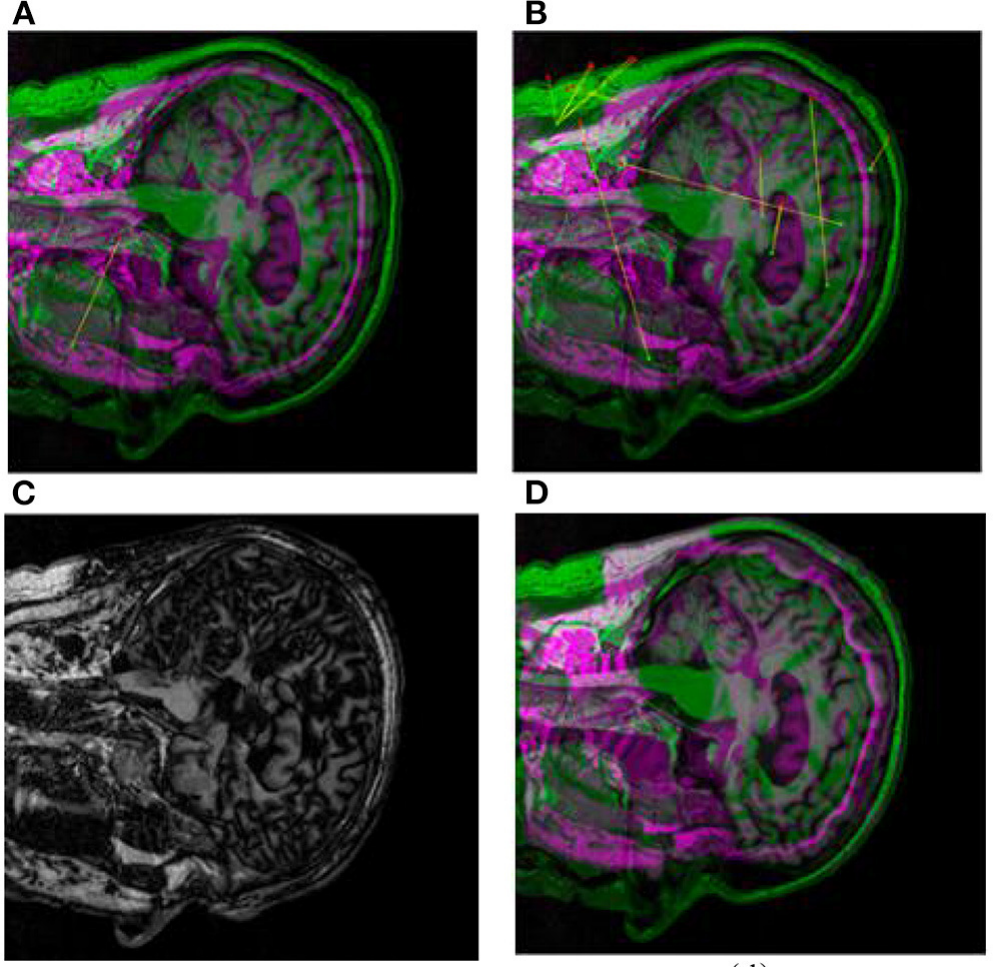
Registration images of brain CT and MRI. (A) shows the pre-processed initial registration image. (B) medical images after multi-modal registration. (C) is affine transformation global rough registration of medical images. (D) represents medical image with high precision registration after non-rigid transformation.
